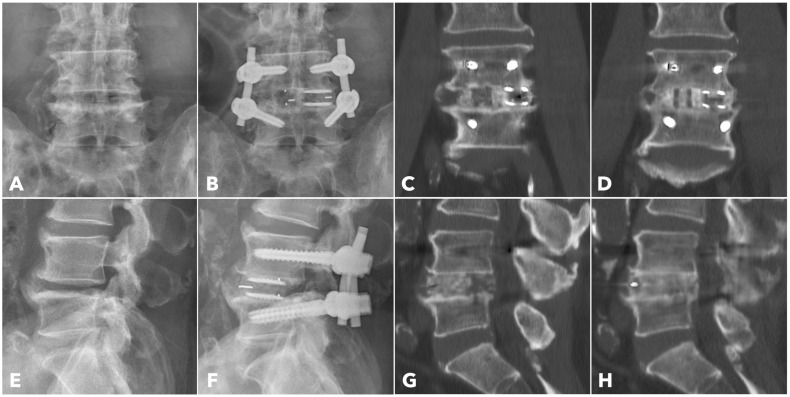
A 67-year-old male patient underwent BETLIF at L4-5 due to recurrent lumbar disc herniation and painful disc degeneration (A,E). The postoperative X-rays showed restoration of the disc height with double cages in the disc space (B,F). The immediate postoperative CT scan demonstrated the cage positions and no violation of the bony endplates (C,G). The 1-year CT scan revealed Bridwell grade I solid fusion with remodeling of the bone graft (D,H).
